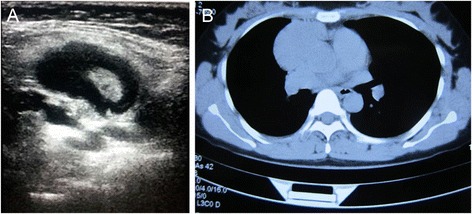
Representative images from ultrasound and CT examinations. a Ultrasound examination showed multiple hypoechoic nodules in the right supraclavicular lymph node. b CT scanning revealed an irregular soft tissue mass (6.2 cm × 3.7 cm) with a clear border in the mediastinum and suppression of the superior vena cava by the mass
